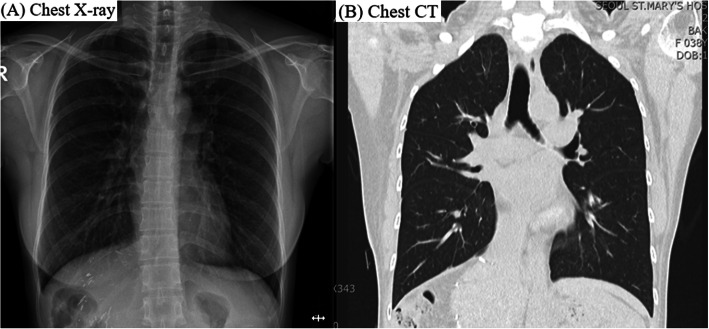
a Chest radiography and b computed tomography (CT) at hospital discharge
